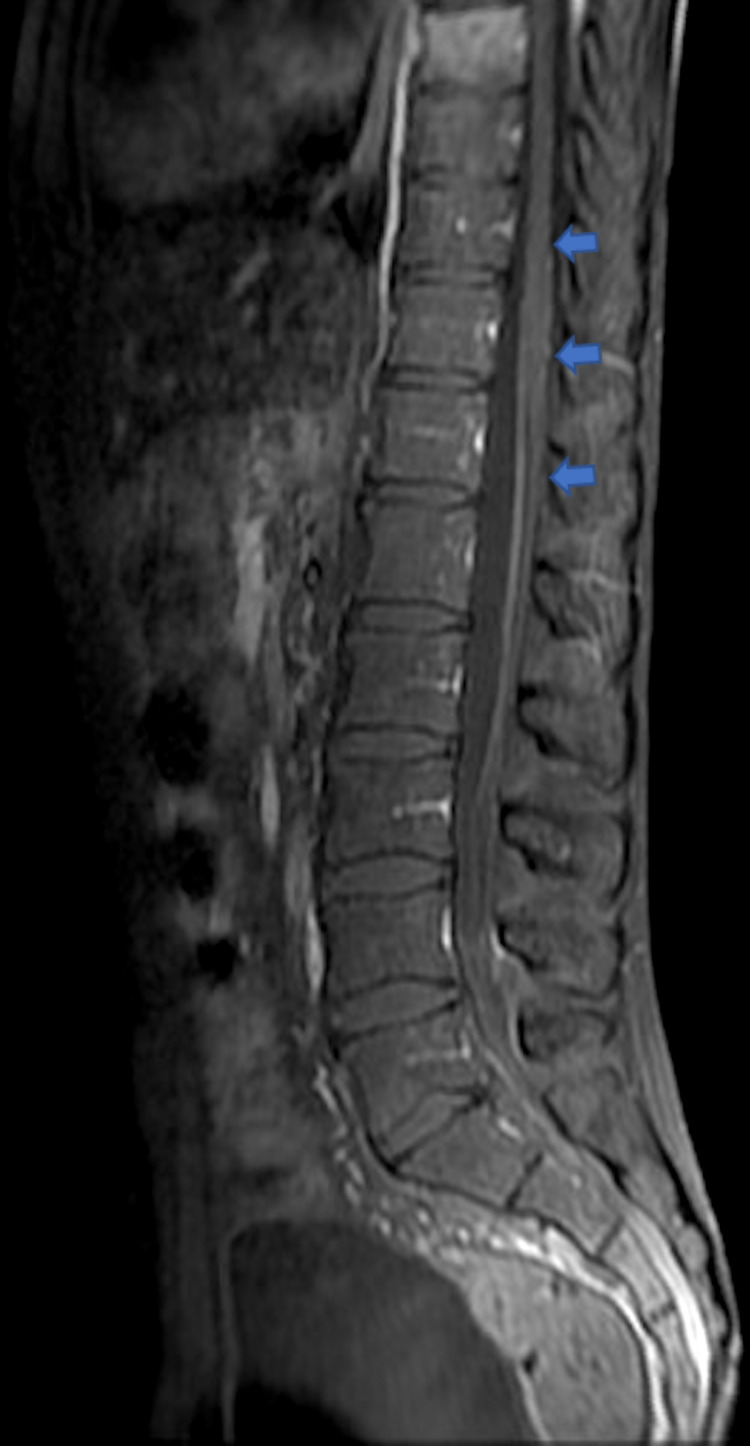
Post-contrast T1-weighted MRI image in sagittal plane demonstrating regression of the leptomeningeal infiltration (shown by arrows) after treatment
